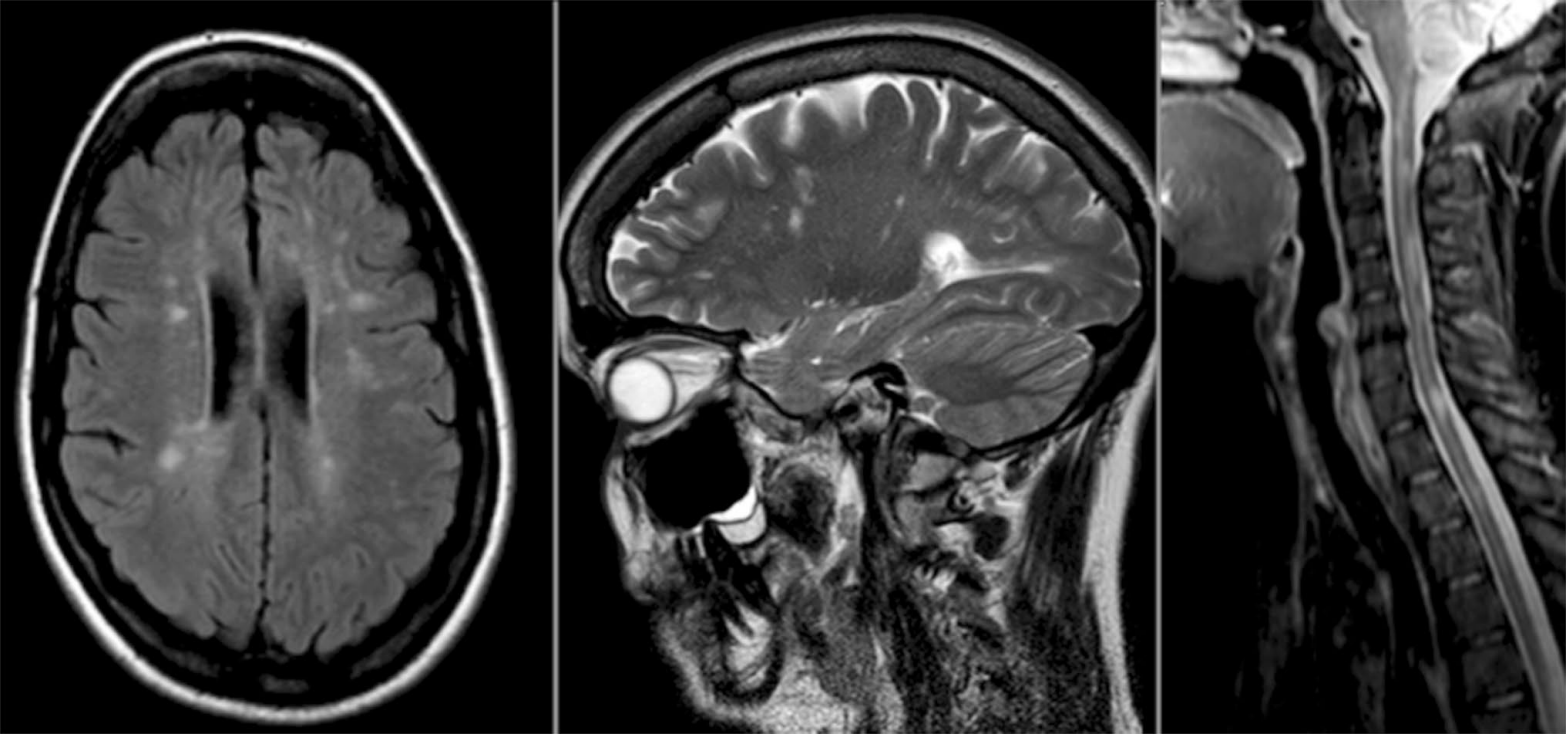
LHON-MS overlap syndrome. T2 MRI images from a 27-year-old woman with episodes of recurrent optic neuritis over a 10-year period associated with partial visual recovery. There are disseminated high-signal changes within the white matter regions of the brain and cervical spinal cord that are consistent with demyelination [105]
